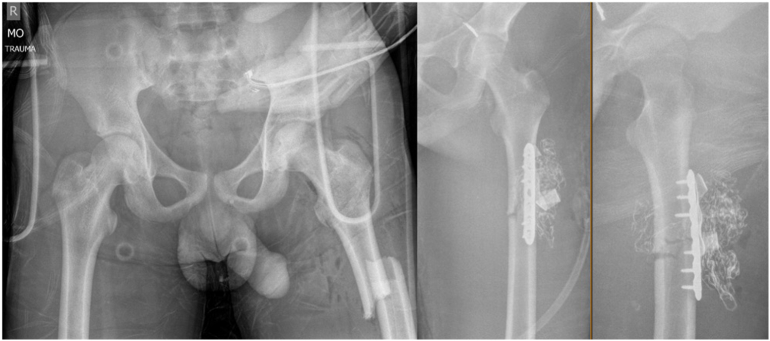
Initial AP pelvis (left), AP left femur (middle), and lateral left femur (right) for case 2. Note the anterior 3.5 mm LCP plate and anterior thigh packing performed as part of initial damage control orthopaedics. The bilateral SI joint dislocations are not well visualized on initial pelvic radiograph.
